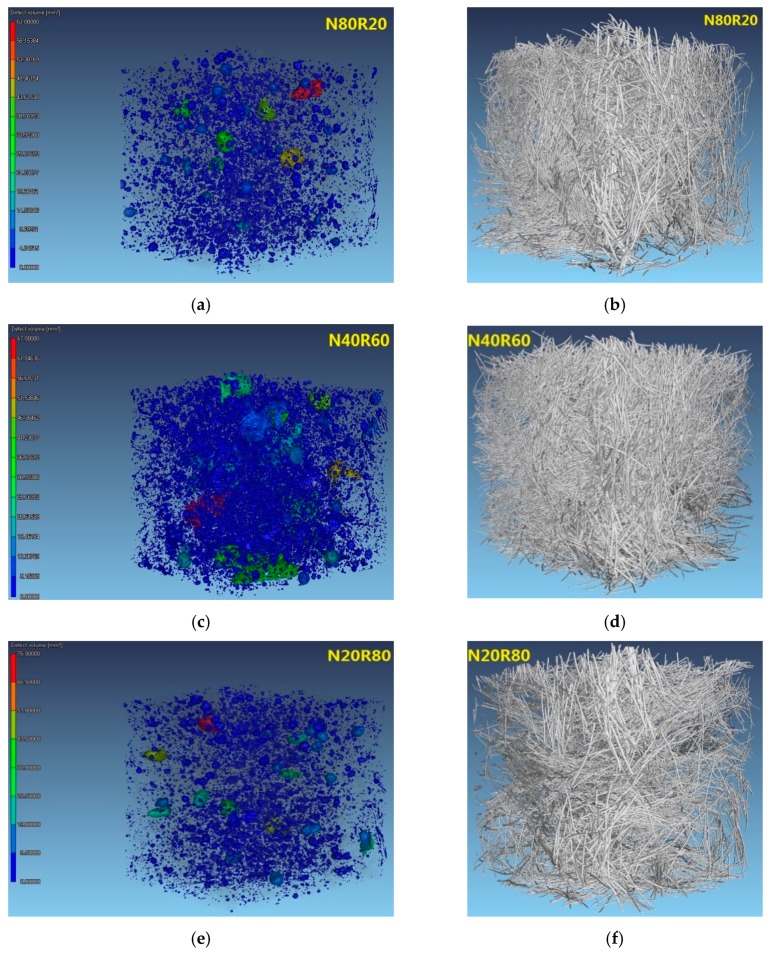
Pores and steel fibres distribution of UHPC by X-CT. (a) Pores distribution of N80R20; (b) Steel fibres distribution of N80R20; (c) Pores distribution of N40R60; (d) Steel fibres distribution of N40R60; (e) Pores distribution of N20R80; (f) Steel fibres distribution of N20R80.
